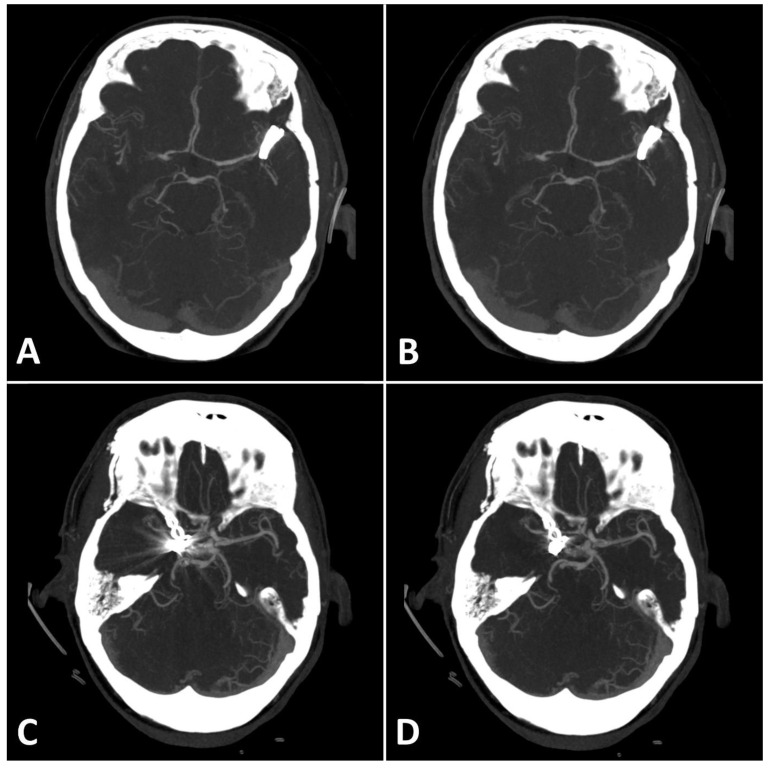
(A,B): Exemplary follow up UHR-CT-angiography in axial plane without (A) and with SEMAR (B) of a 74-year-old female with an intracranial clip in the left middle cerebral artery. (C,D): Exemplary follow up UHR-CT-angiography in axial plane without (C) and with SEMAR (D) of a 63-year-old female with an intracranial clip in the right internal carotid artery. All images were reconstructed as MIP (Maximum Intensity Projection) with 12.5 mm slice thickness and demonstrate the effects of SEMAR on images with clip-artifacts.
